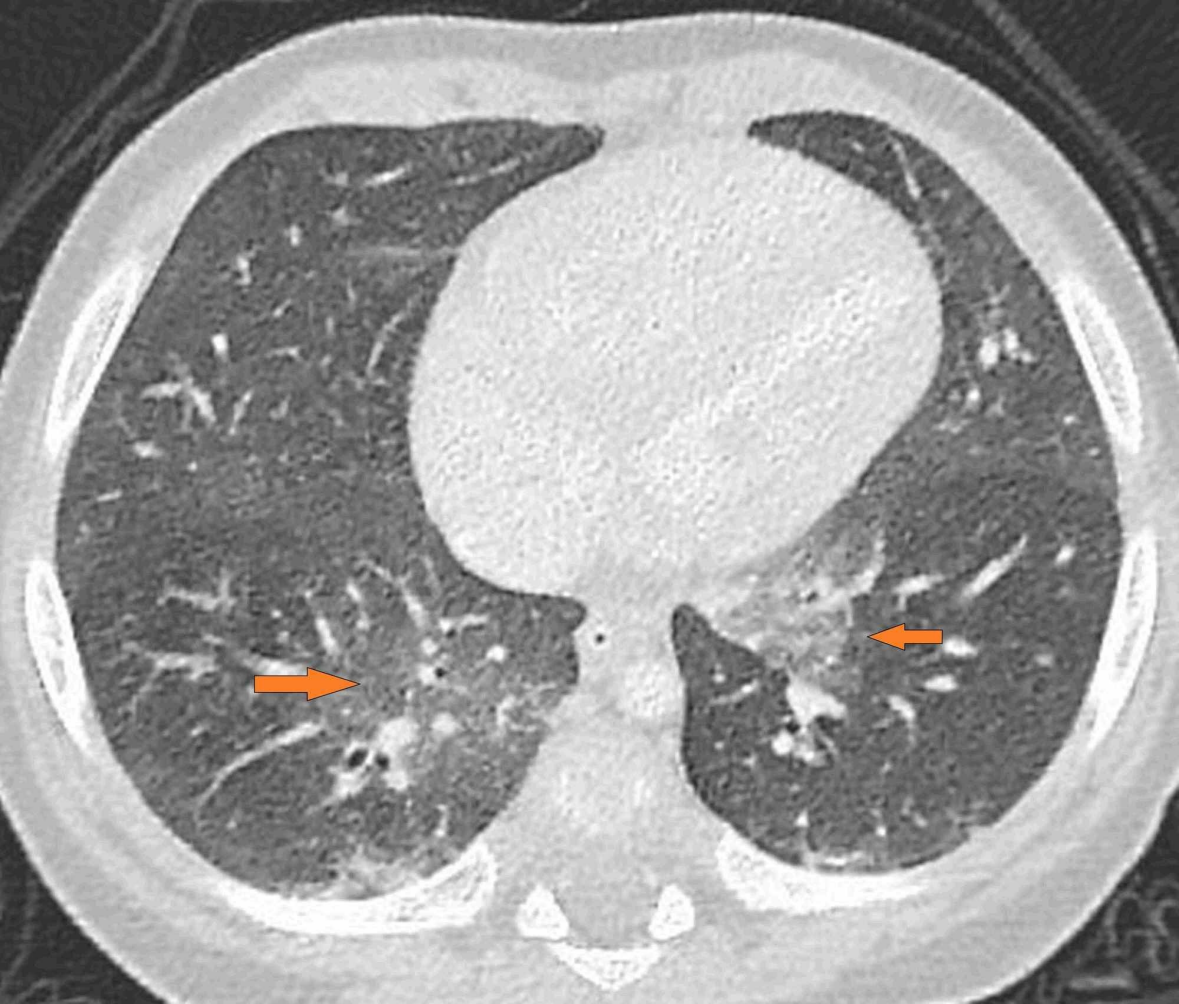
Axial HRCT section of the lung at the level of the lower lobe Arrows on both sides show the geographic ground-glass opacities. HRCT, high-resolution computed tomography
